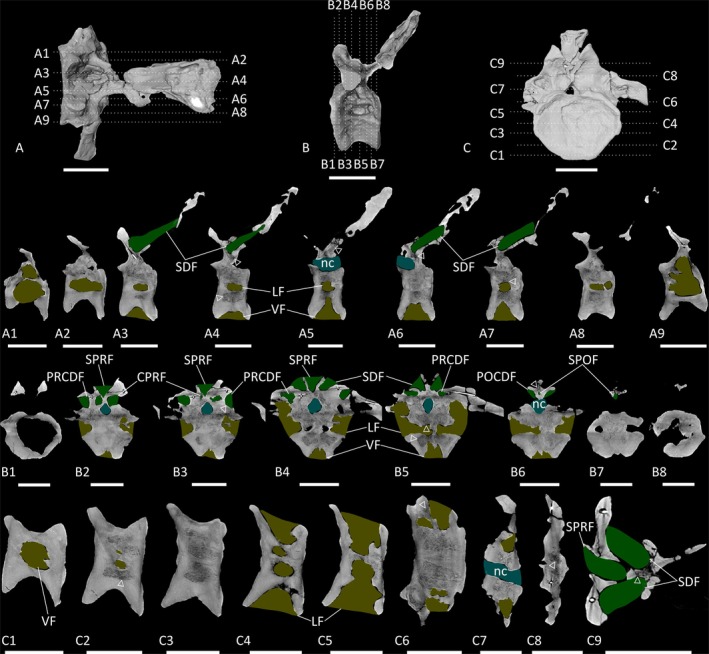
CT scan of *Tornieria africana* anterior caudal vertebra MB.R. 2956.1. (A–C) 3D rendering (A) dorsal view, (B) left lateral view, (C) anterior view; (A1–A9) CT scan slices in parasagittal cross‐section, (B1–B8) CT scan slices in transverse cross‐section, (C1–C9) CT scan slices in horizontal cross‐section. CPRF, centroprezygapophyseal fossa; LF, lateral fossa; nc, neural canal; POCDF, postzygapophyseal centrodiapophyseal fossa; PRCDF, prezygapophyseal centrodiapophyseal fossa; SDF, spinodiapophyseal fossa; SPOF, spinopostzygapophyseal fossa; SPRF, spinoprezygapophyseal fossa; VF, ventral fossa. Key: Blue highlight, neural canal; green highlight, neural spine fossa; yellow highlight, centrum fossa; white arrow, pneumatic bone texture. Scale bars = 100 mm.
